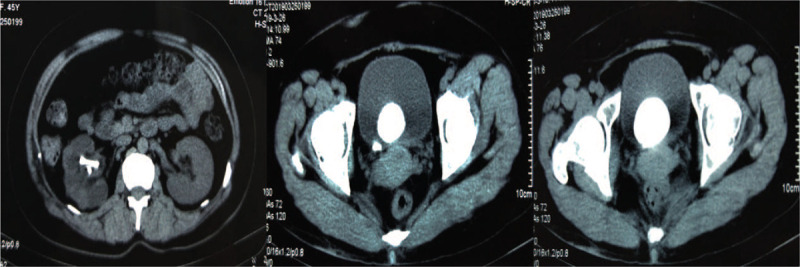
Giant bladder stone, an encrusted D-J stent, normal left kidney, and right hydronephrosis on CT scans. CT = computed tomography, D-J stent = double-J stent.
